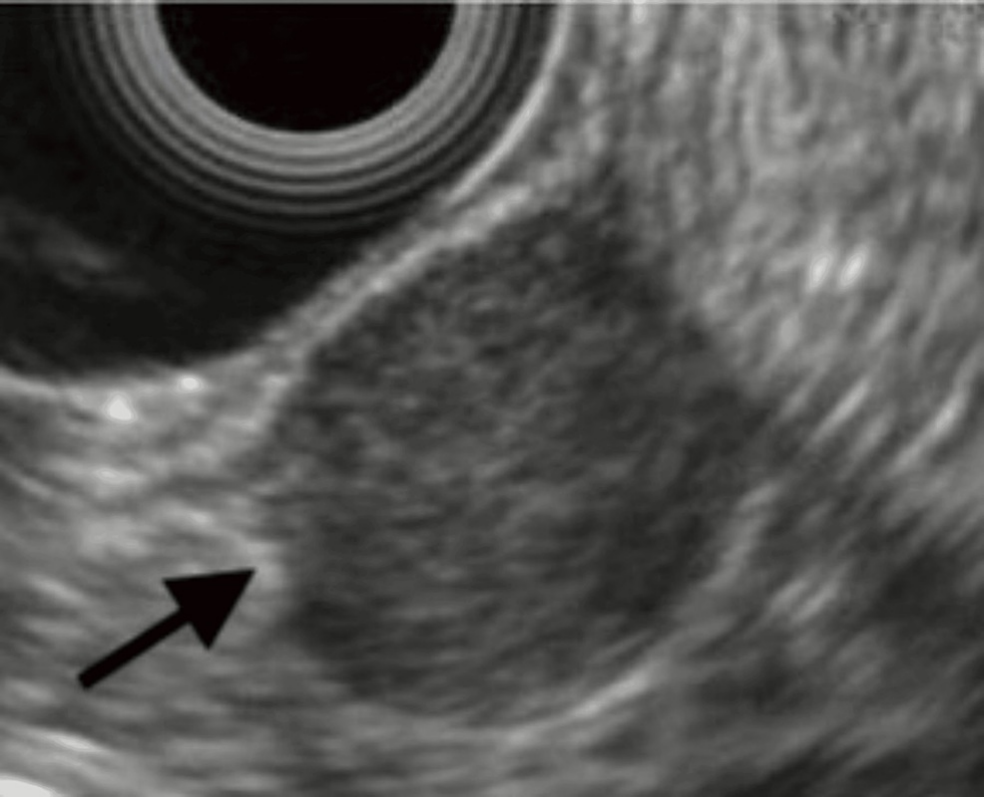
EUS image showing an 18.2 mm x 17.9 mm hypoechoic lesion at the level of first portion duodenal structure arising from the muscularis propria of the duodenal wall (arrowed). FNA biopsy of the lesion confirmed the recurrence of the patient’s urothelial carcinoma. EUS, endoscopic ultrasound; FNA, fine-needle aspiration
